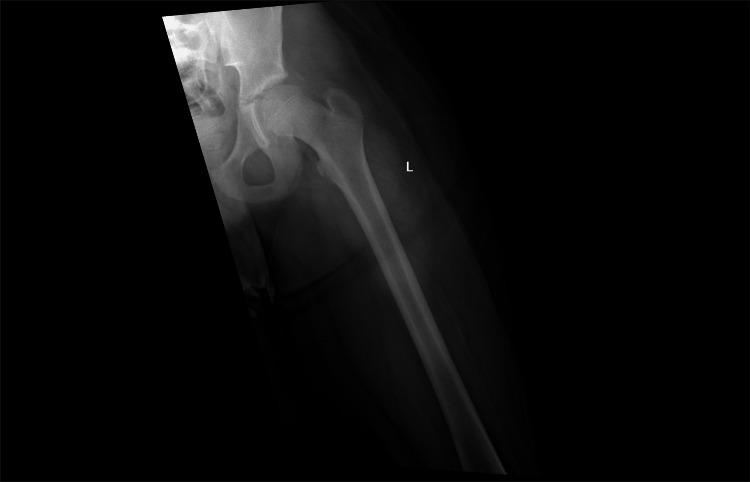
AP X-ray of the left hip
